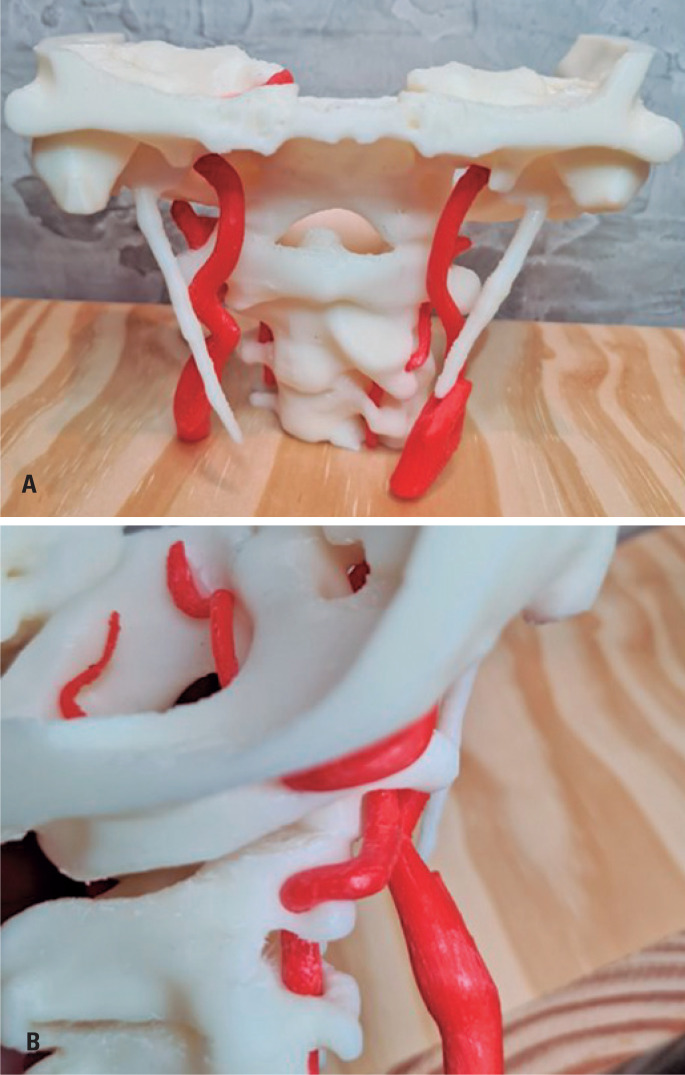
Anterior coronal view (A) and left lateral view (B) of the 3D-printed model of the left rotated head and neck Computed tomography acquisition showing the relation between the main cervical arteries with regional bone structures. Right lateral view (B) focuses on showing the relation between the left C2 transverse process and the V2/V3 transition of the right vertebral artery
